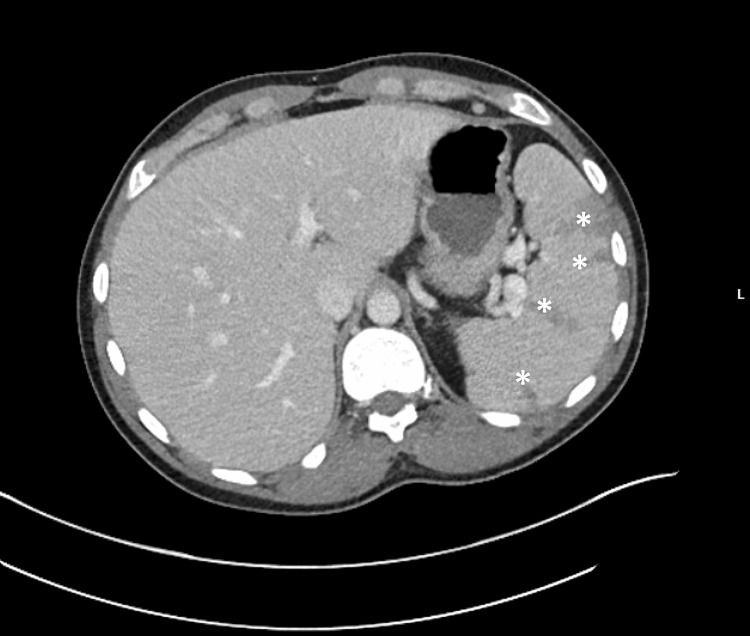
CT abdomen demonstrating splenic infarcts (asterisks). CT shows multiple wedge-shaped areas of decreased enhancement in the spleen, consistent with splenic infarcts.
